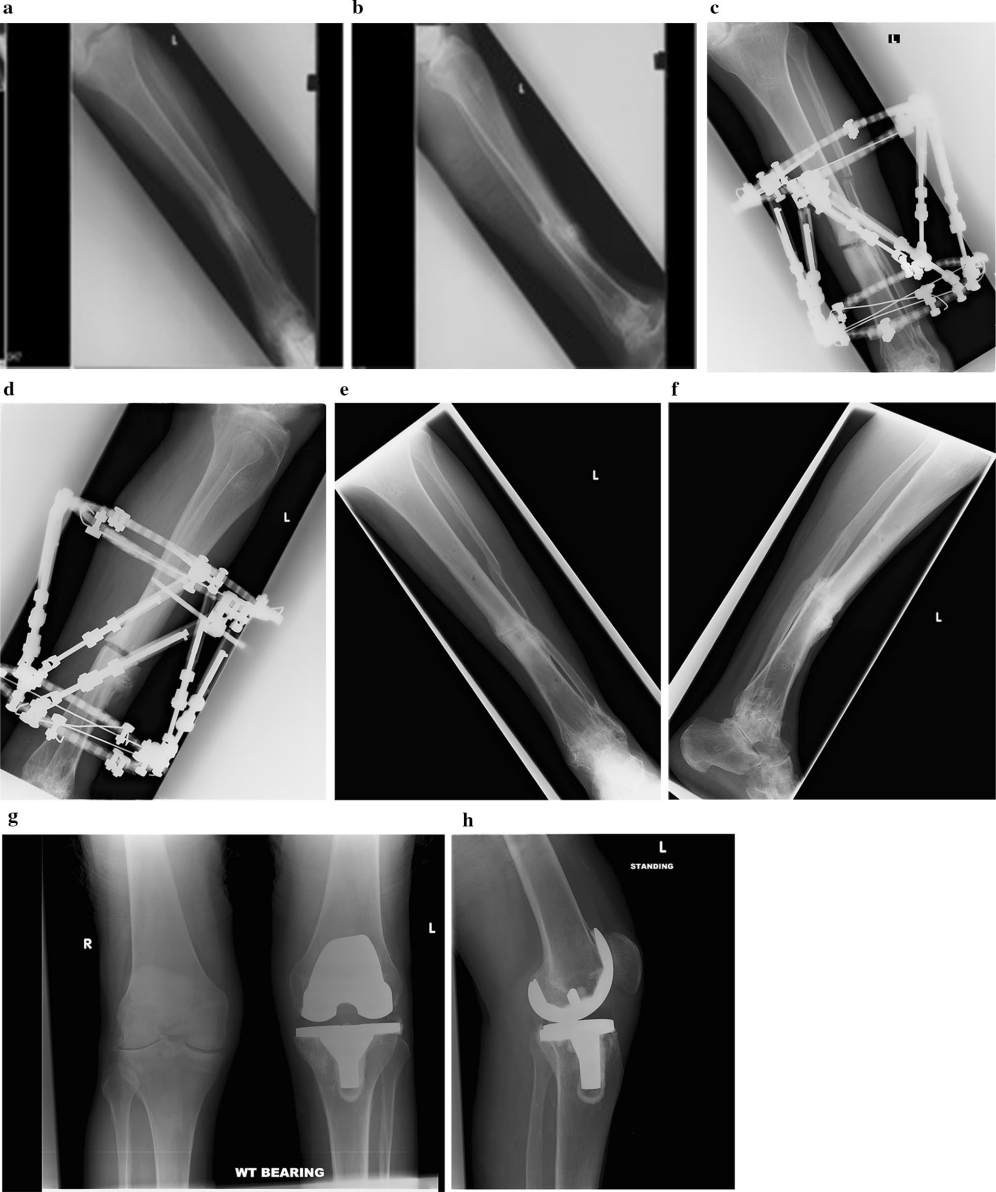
Case 2: a, b Orthogonal radiographs of tibial malunion deformity used for deformity correction planning. c, d Orthogonal radiographs showing Taylor spatial frame application with corrective tibial osteotomy away form the CORA. e, f Orthogonal radiograph showing realignment of the mechanical axis and healing of the osteotomy site with an established ankle arthrodesis. g, h Orthogonal radiographs showing total knee replacement in situ performed as a staged procedure
